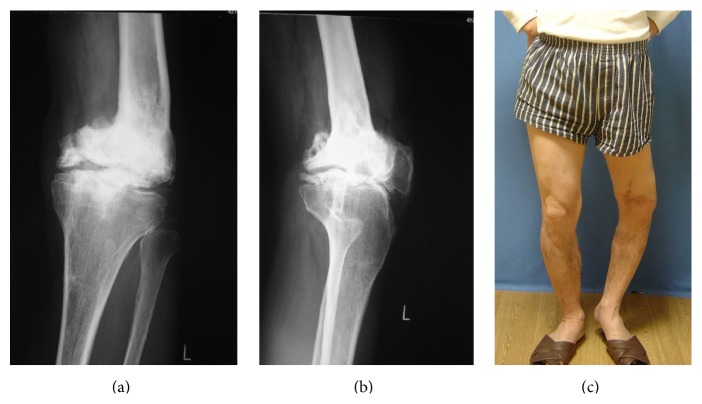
Preoperative condition. (a, b) Anteroposterior and lateral X-P (plain X-ray or roentgenography) of the knee showing partial pseudarthrosis of the knee and varus deformity of the joint. (c) Clinical photo of the patient shows marked bowing.
